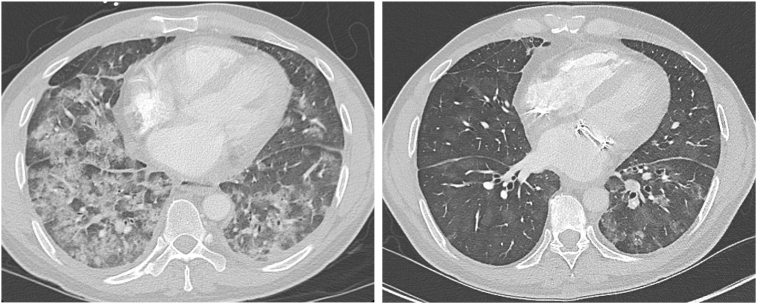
Chest CT, axial display with contrast (lung windows), concerning for DAH (*left*) from MS and its subsequent resolution after MV replacement (*right*).
